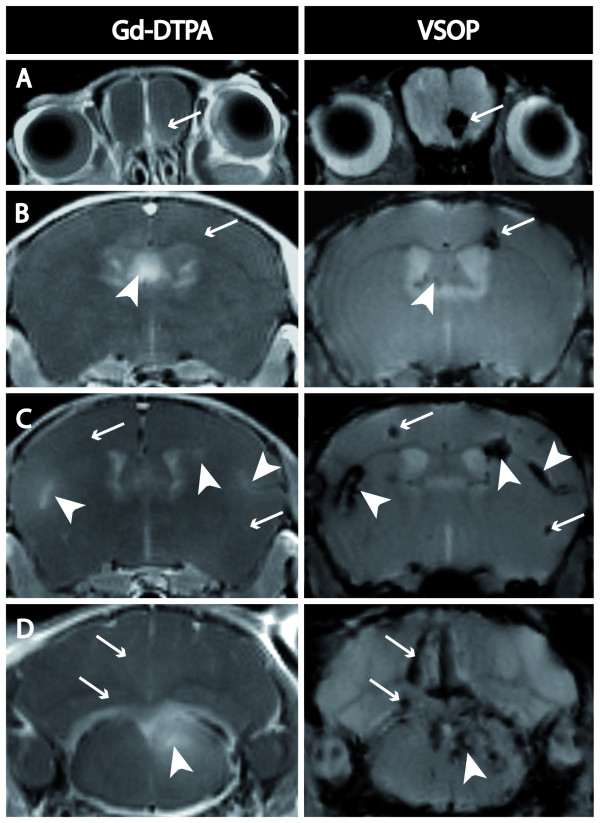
Comparison of contrast enhancing lesions. Comparison of coronal T1-weighted images immediately after Gd-DTPA administration versus T2*-weighted images 24 h after VSOP application. The majority of the lesions enhanced both, Gd-DTPA and VSOP, although shape differences within individual lesions were apparent (arrow heads). Furthermore, several plaques enhanced exclusively VSOP, but not Gd-DTPA (small arrows).
